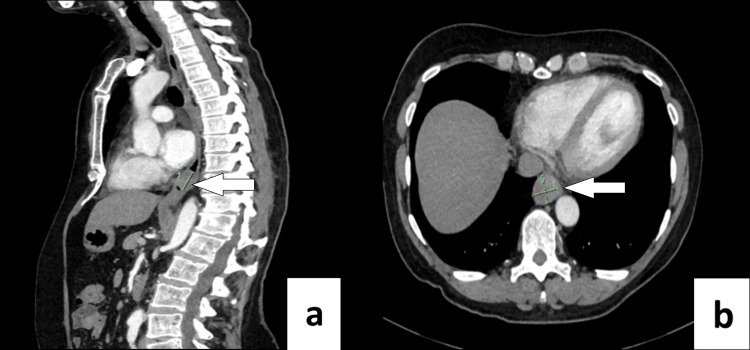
Pre-operative Computed Tomography (CT) scan Pre-operative Computed Tomography (CT) scan (a. sagittal, b. axial views) of a distal oesophageal mass likely representing a schwannoma, measuring approximately 2.2 cm x 2.4 cm transaxially and approximately 2.4 cm in craniocaudal length. White arrows indicate distal oesophageal mass.
